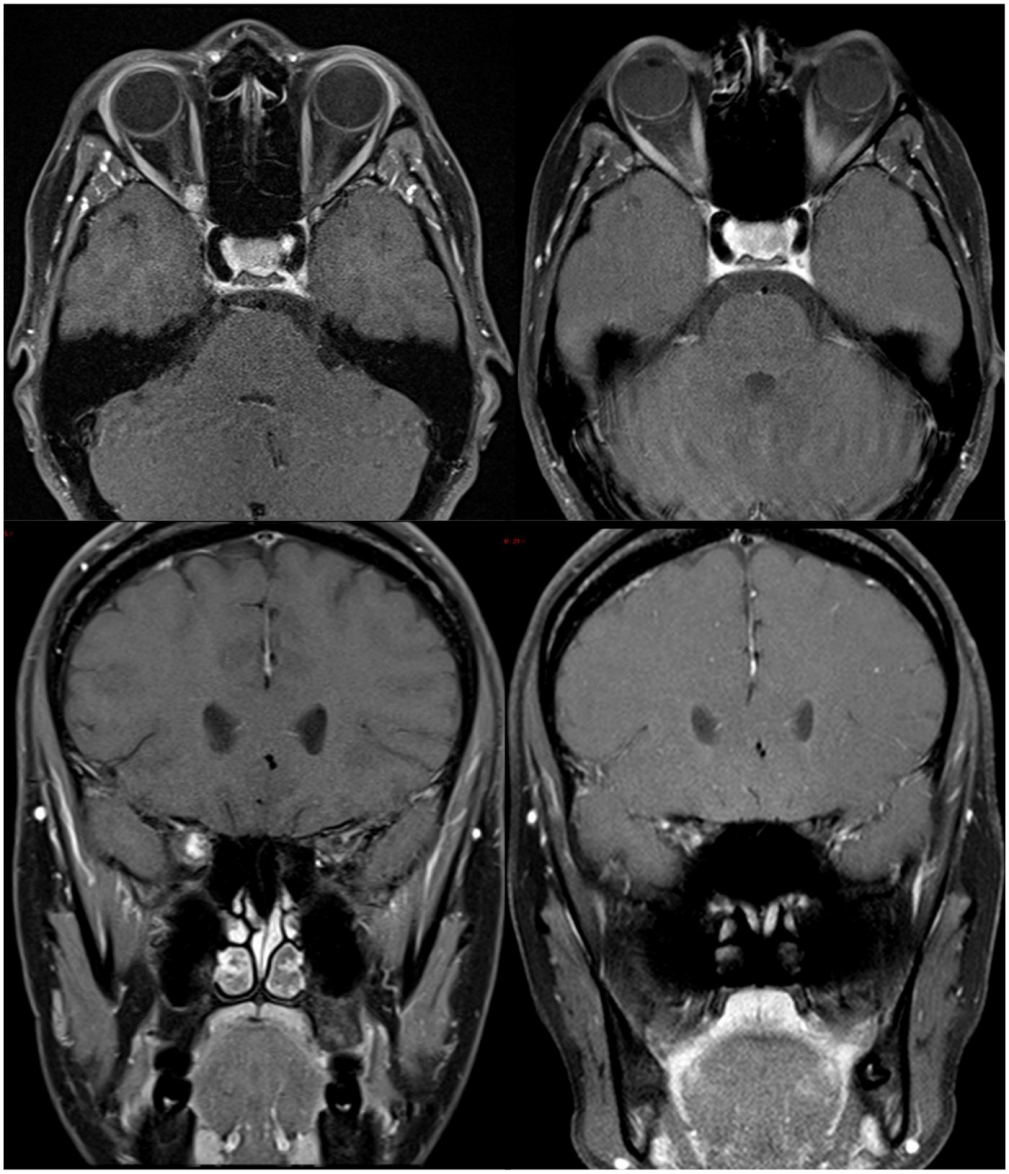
Patient 5. Twenty-seven-years-old female experiencing blurred vision. (Top left) Pre-treatment axial T1 weighted fat-suppressed post-contrast MR image showing a well-defined, homogeneously enhancing intraconal mass at the right orbital apex. (Top right) Post-radiotherapy axial T1 weighted fat-suppressed post-contrast MR. (Bottom left) Pre-treatment coronal T1 weighted fat-suppressed post-contrast MR. (Bottom right) Post-radiotherapy MR demonstrates reduction in size of the intraconal right orbital apex mass.
